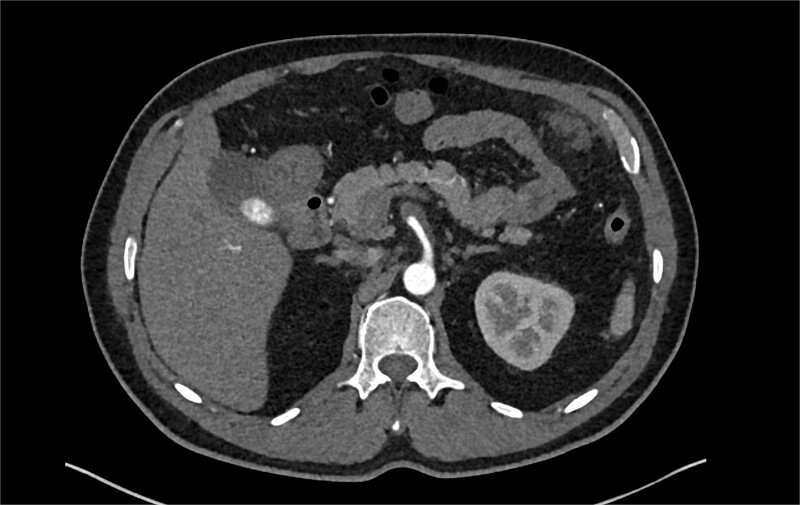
CTA demonstrating a more extensive SMA dissection extending into branch vessels. New hemoperitoneum most pronounced in the left abdomen with no active extravasation noted but probably arises from the left mesentery.
